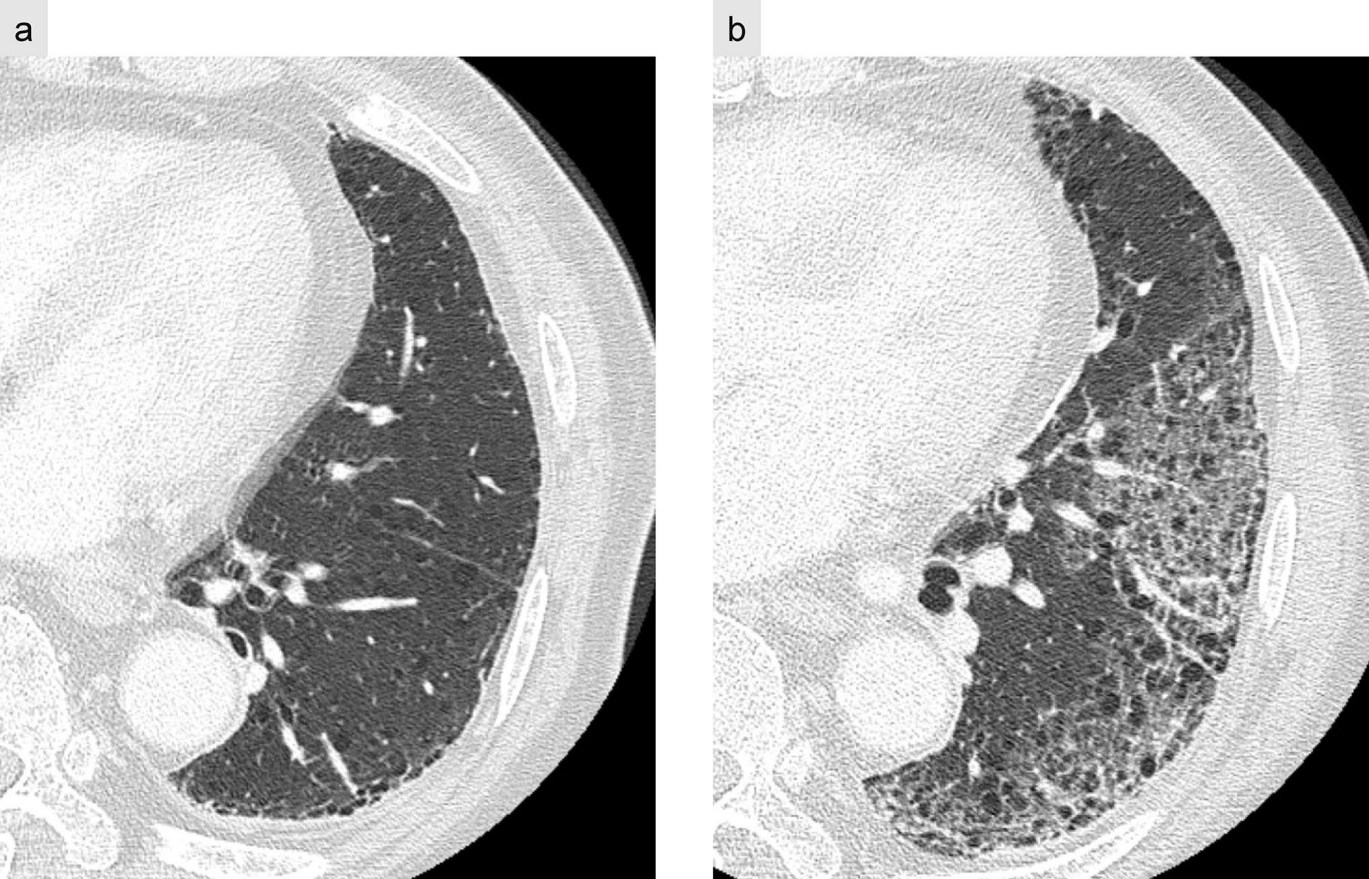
Postoperative acute exacerbation of idiopathic pulmonary fibrosis. Preoperative CT showed interstitial lung disease with the usual interstitial pneumonia pattern, including honeycombing (a). Four days after right upper lobectomy, the patient developed significant hypoxemia, prompting CT imaging. High-resolution CT reveals newly developed diffuse ground-glass and reticular opacities (b)
